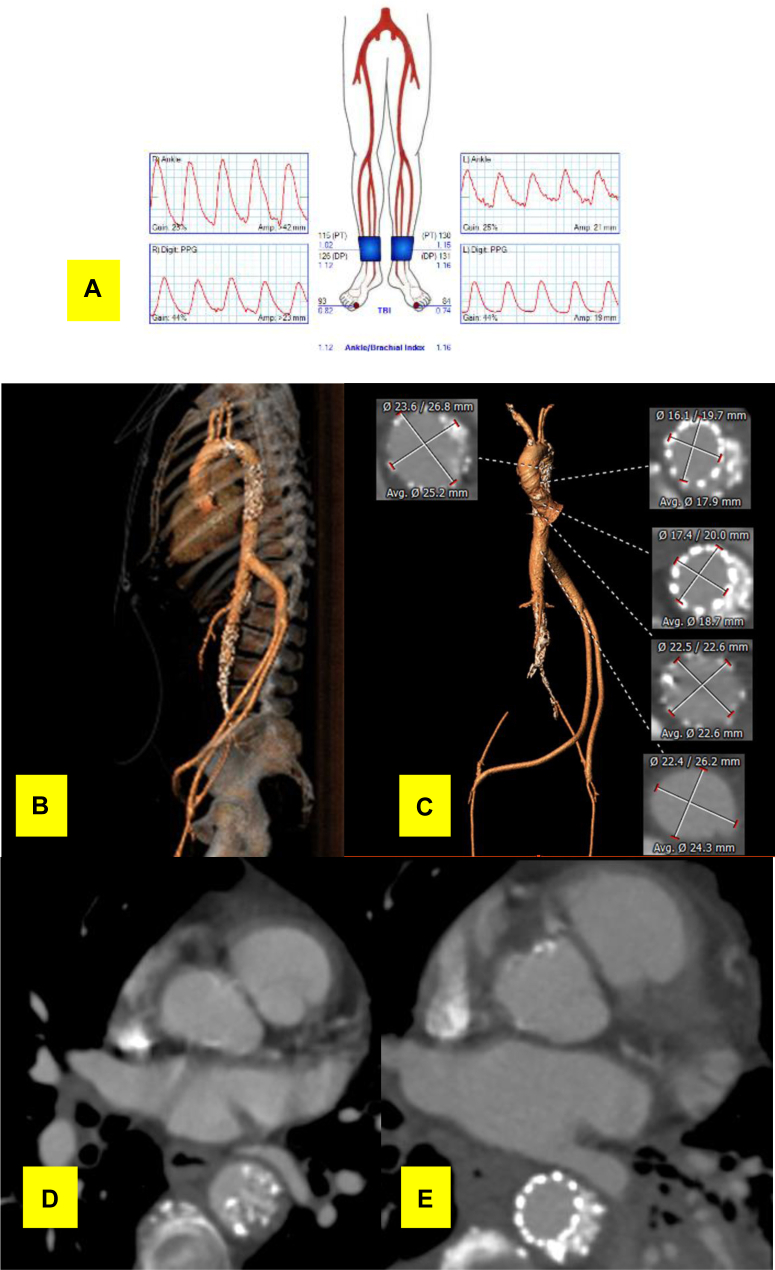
Postoperative follow-up ankle brachial index (A), computed tomography angiogram (CTA; B and C), and the stenotic thoracic aorta pre- (D) and postoperatively (E). *Amp.,* Amplitude; *Avg.,* average; *L,* left; *R,* right.
